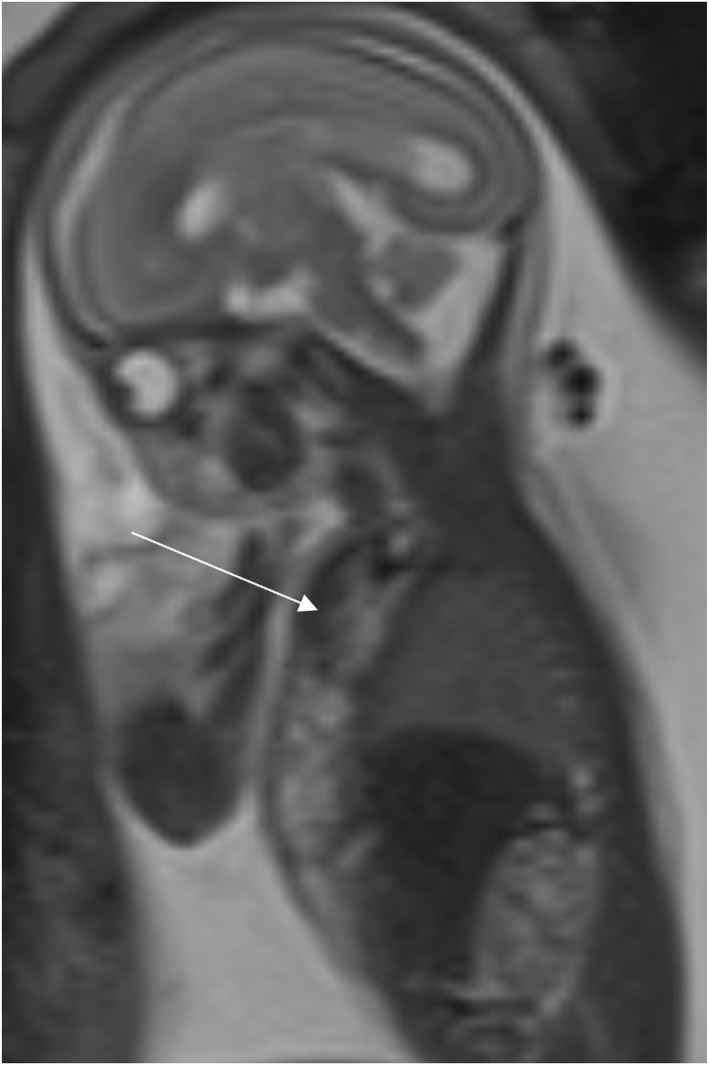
Fetal MRI image of a Kaposiform haemangioendothelioma. T2 haste sagittal image of a 22 weeks fetus with extensive subcutaneous mixed venous and lymphatic malformation and extension into the neck and anterior aspect of the mediastinum consistent with the known lymphatic drainage pattern.
